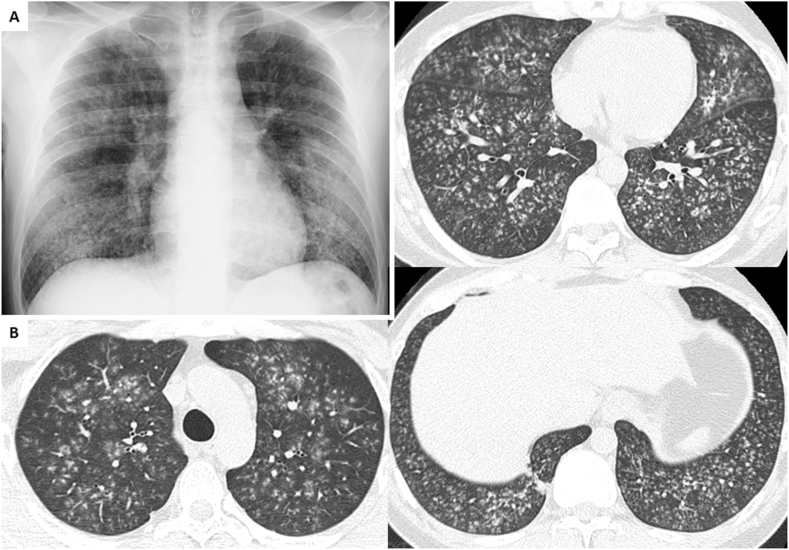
Chest radiography on admission, showing diffuse reticulonodular abnormalities in both lung fields (A). Chest computed tomography on admission showing multiple centrilobular small nodules and ground-glass appearances in both lungs (B).
